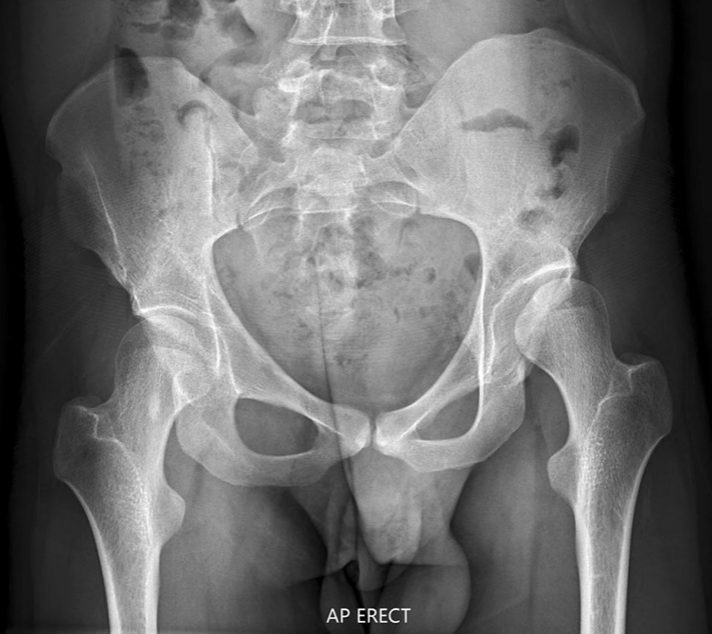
Anteroposterior (*AP*) pelvic radiograph of a young adult aged 21 years, GMFCS level III at the time of transition. Previous surgical history included bilateral adductor releases as part of single-event multilevel surgery to improve gait and functioning. At the time of transition there were no complaints of pain, and the patient walked well with a Functional Mobility Scale score of 5, 5, 5. The right hip is grade II according to the MCPHCS and will probably function well in adult life. However, the left hip is subluxated, dysplastic and is MCPHCS grade IV. Advice was given to continue with hip surveillance and to consider reconstructive surgery at the onset of any symptoms. Referral to an orthopaedic surgeon with an interest in hip dysplasia in young adults was also made
